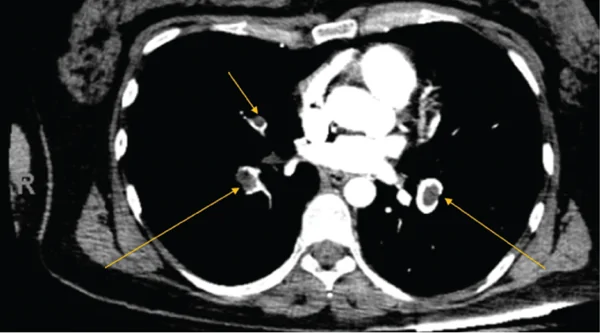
CTA chest pulmonary embolism with IV contrast revealed bilateral PE (yellow arrows).
